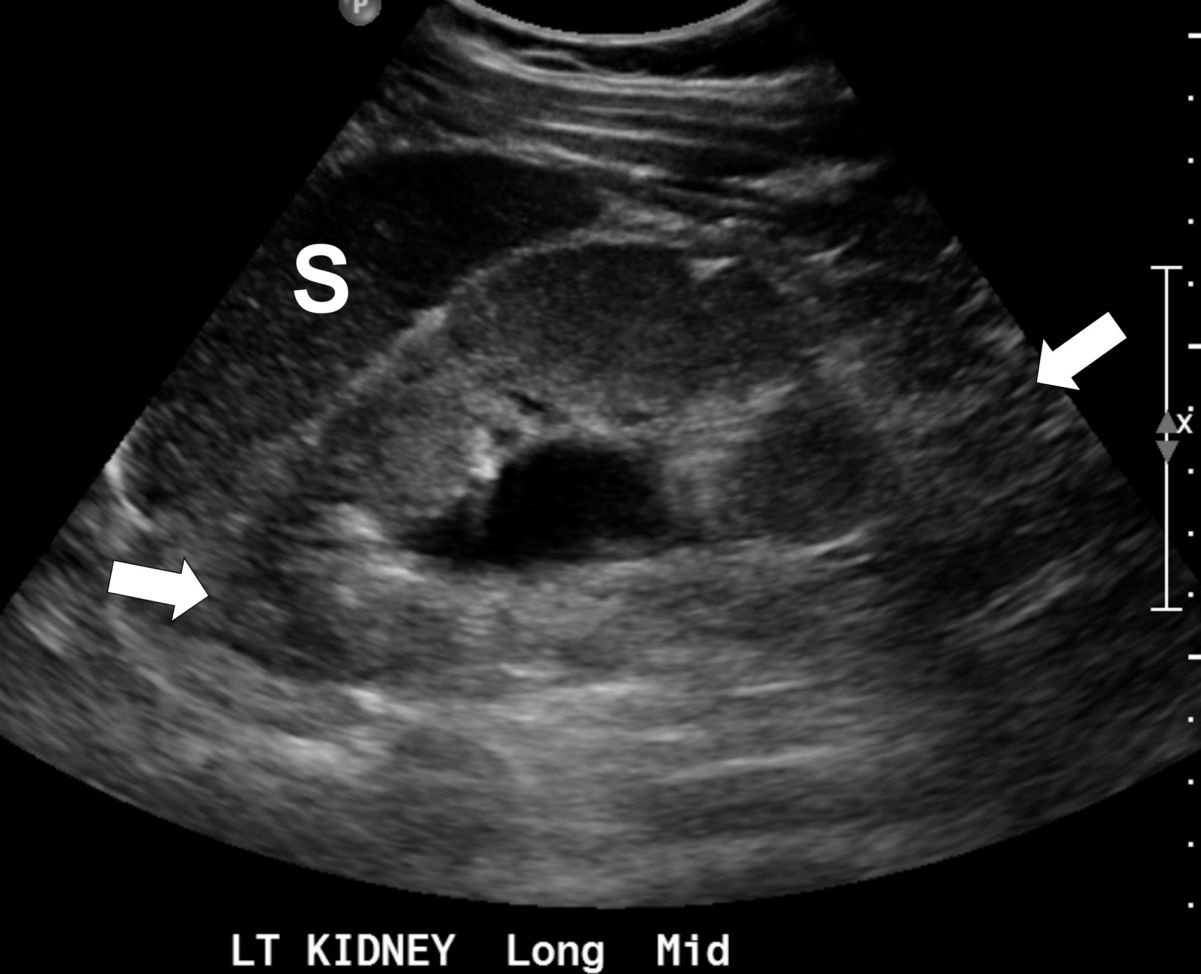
Longitudinal ultrasound of the left kidney Longitudinal ultrasound image of the left kidney (arrows) shows a top normal size kidney with mild pelviectasis, and mild, diffuse increase in parenchymal echogenicity with poor corticomedullary differentiation. The renal parenchyma appears mildly increased in echogenicity when compared to the adjacent splenic (S) parenchyma.
